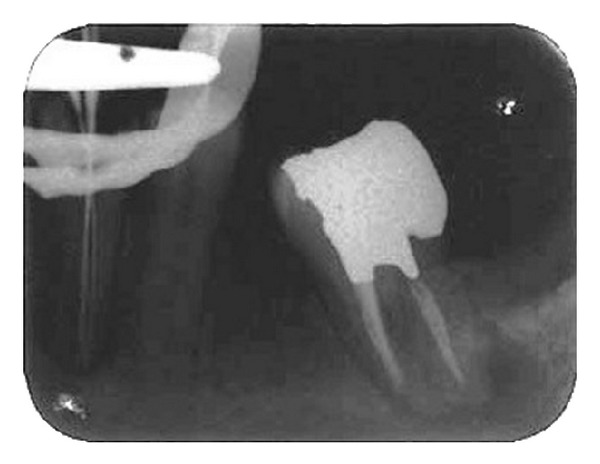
Working length radiograph confirming three root canal orifices.
